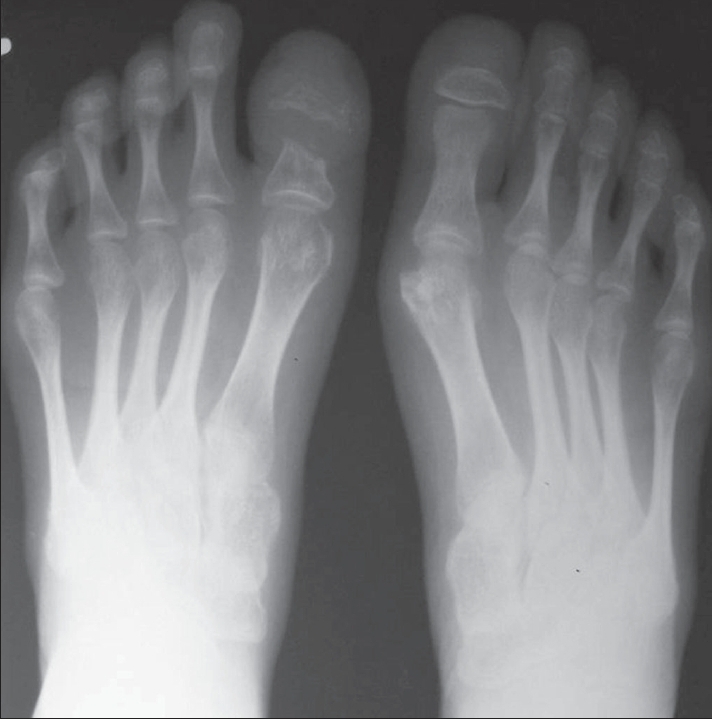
A frontal foot radiograph shows acro-osteolysis of the distal phalanges of both great toes, with resorption of the proximal phalanx of the right great toe
